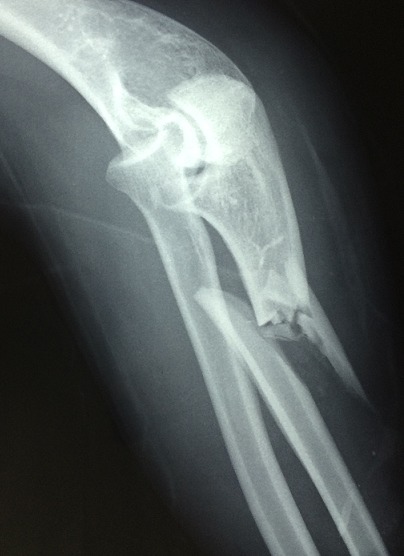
Radiography of monteggia fracture
